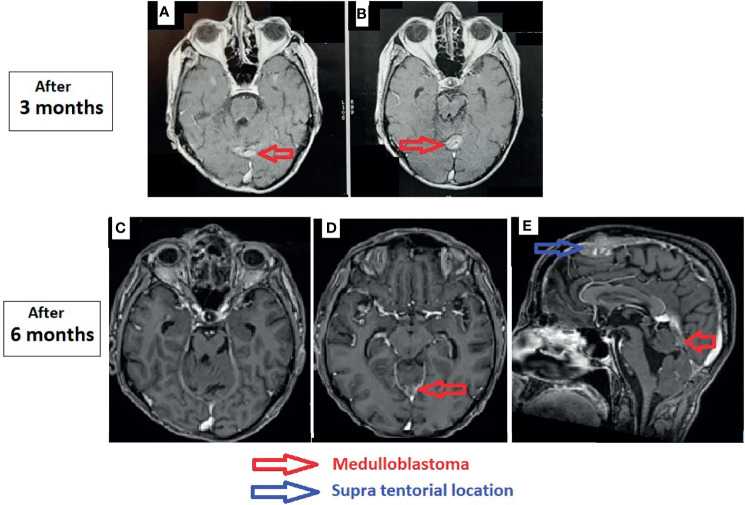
Control magnetic resonance imaging of the brain. (A, B) After 3 months: axial T1-weighted post-gadolinium. (C–E) After 6 months: axial T1-weighted post-gadolinium (C, D) and sagittal T1-weighted post-gadolinium (E).
